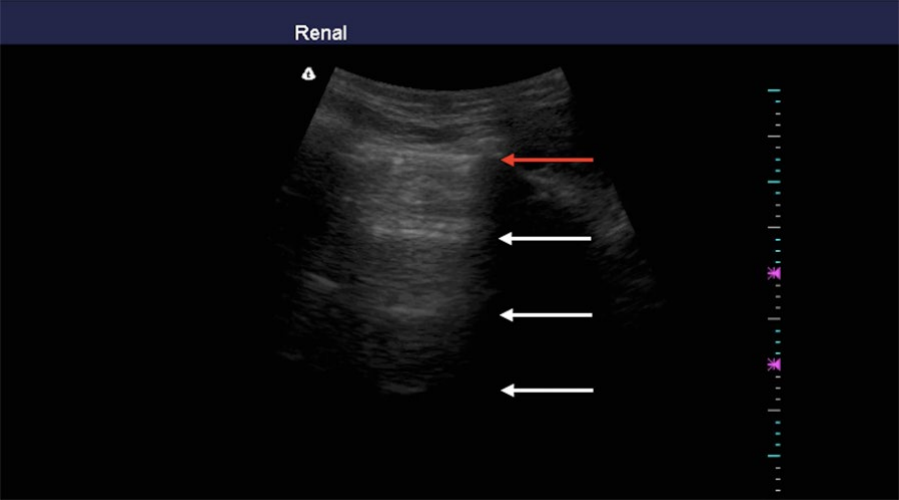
Sonographic appearance of an aerated lung scan in longitudinal view. Red arrow indicates pleural line; white arrows indicate A-lines
